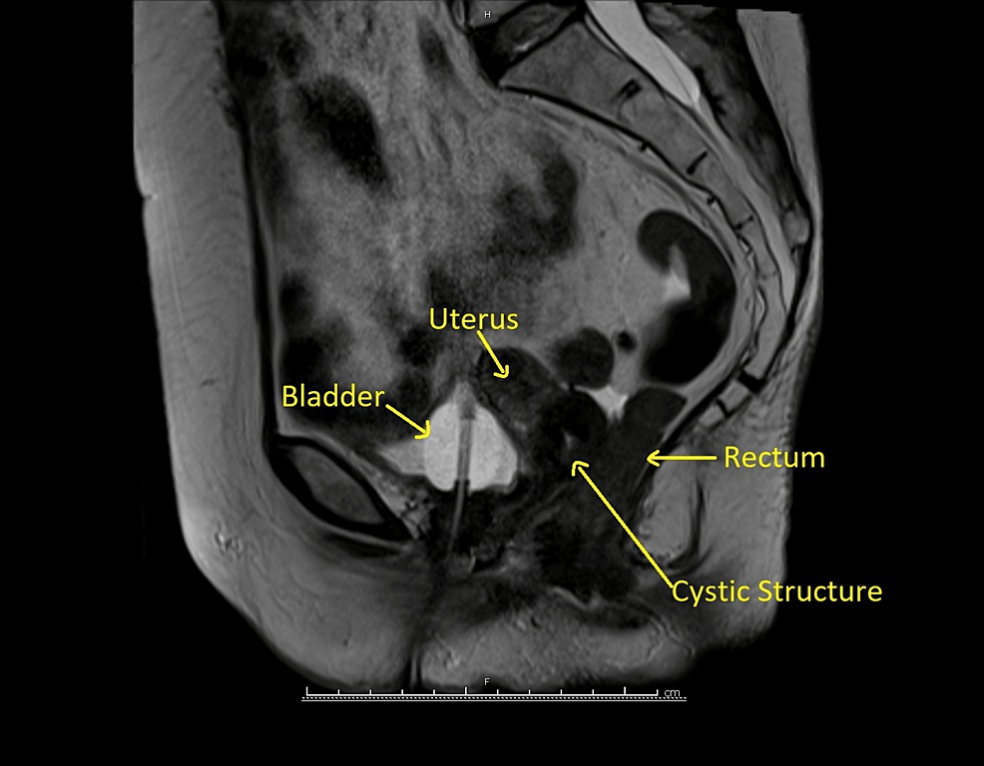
MRI pelvis: midsagittal view
